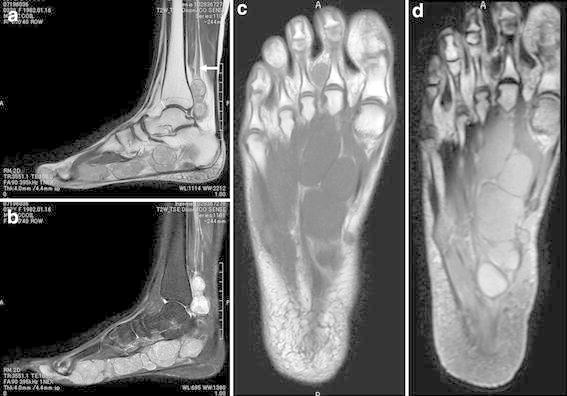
Magnetic resonance imaging of the right foot and ankle. a Sagittal T2-weighted image showing multiple hyperintense lesions along the course of the posterior tibial (*white arrow*) and medial plantar nerves. b Sagittal T2-weighted image with fat suppression demonstrating multiple nodular lesions with heterogeneous high signal intensity. c Coronal T1-weighted image showing the lesions with iso-signal intensity relative to adjacent muscle. d Coronal contrast-enhanced T1-weighted image with fat suppression revealing mild to moderate enhancement of the lesions.
